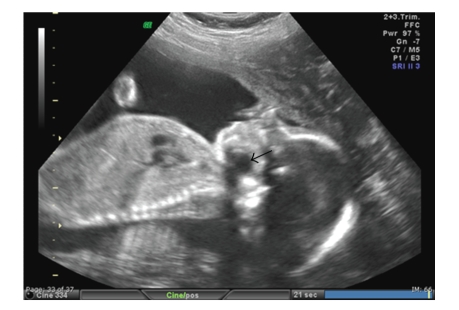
Ultrasound image at 26-week gestation demonstrating oropharyngeal cyst (black arrow).
